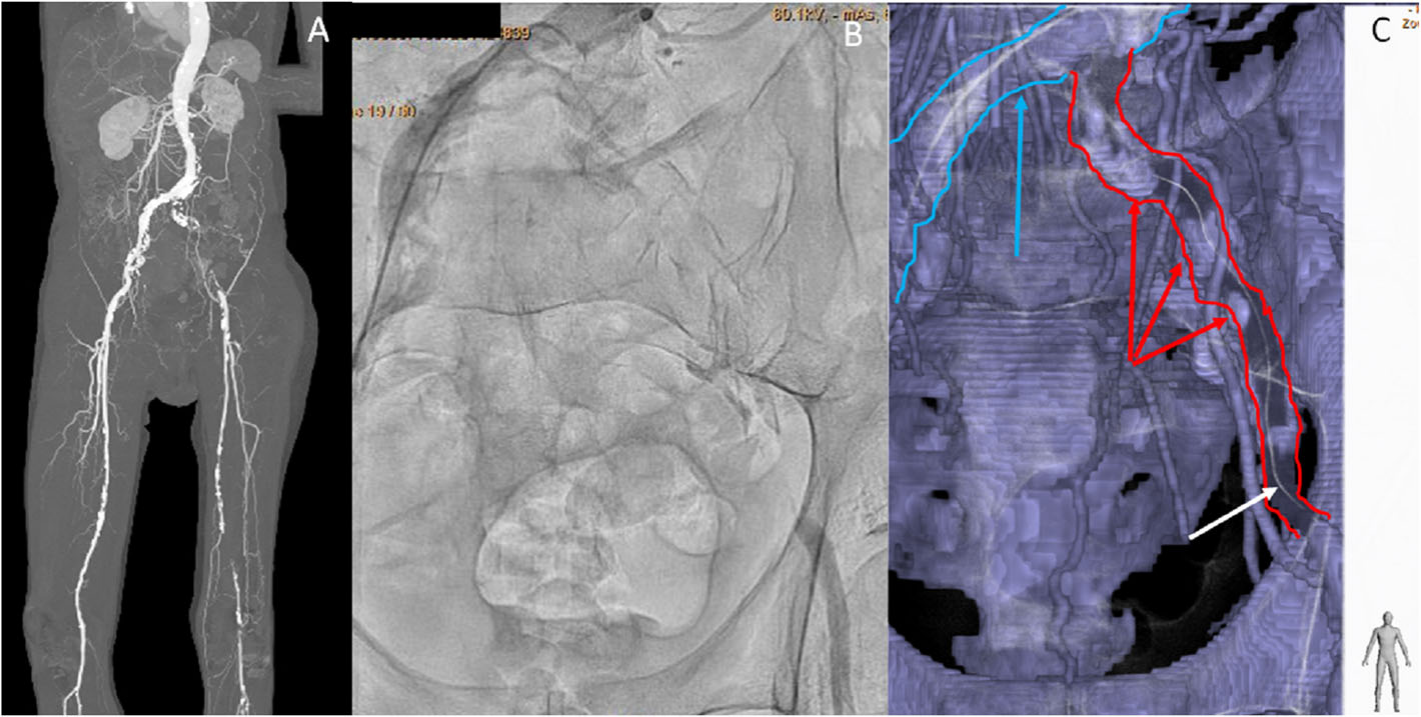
a: Pre procedural enhanced CT revealed total occlusion from the ostium of the left common iliac artery (CIA) to the distal end of the external iliac artery (EIA) and total occlusion of the left superficial femoral artery. b: Bidirectional control angiography revealed total occlusion from the ostium of the left CIA to the distal aspect of the left EIA. c: This is CTf3D-RM (blue arrow showed opened aorta and right CIA, red arrow showed virtual occluded vessels, white arrow showed guidewire). The control angiography and CTf3D-RM images matched well
